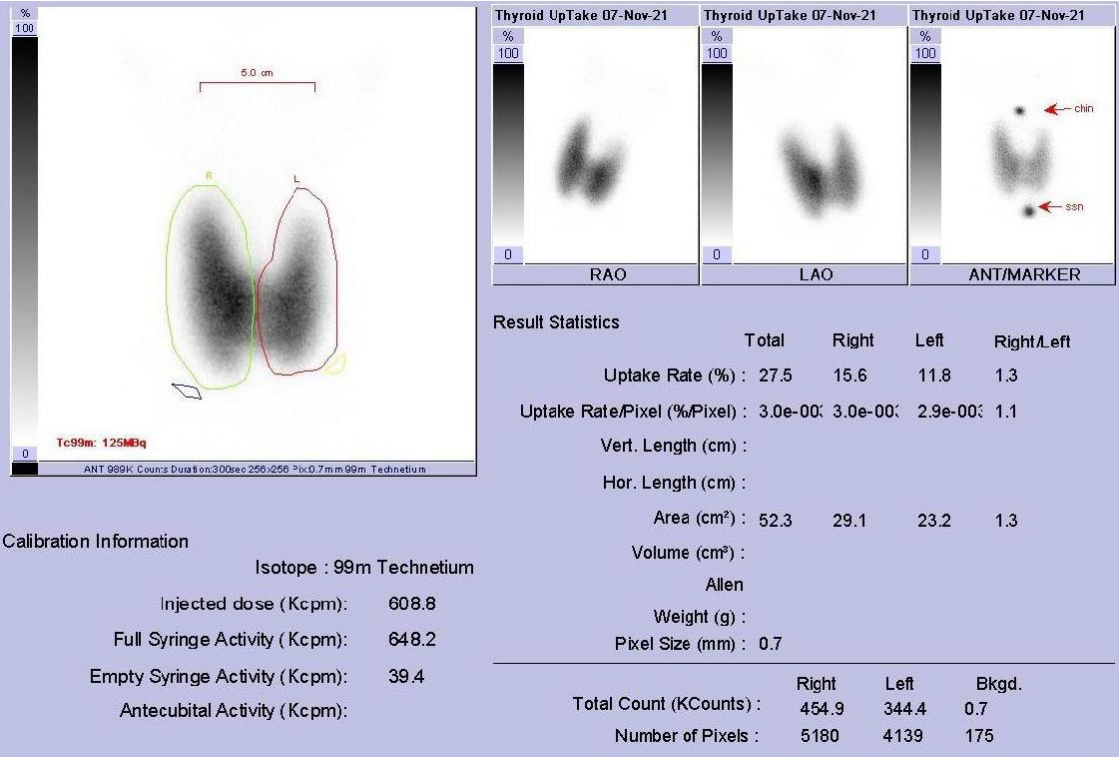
Tc-99m pertechnetate thyroid scintigraphy (thyroid scan), reveals diffusely increased radiotracer uptake in bilateral thyroid lobes, with suppression of the background activity. Global thyroid uptake was 27.5 %
